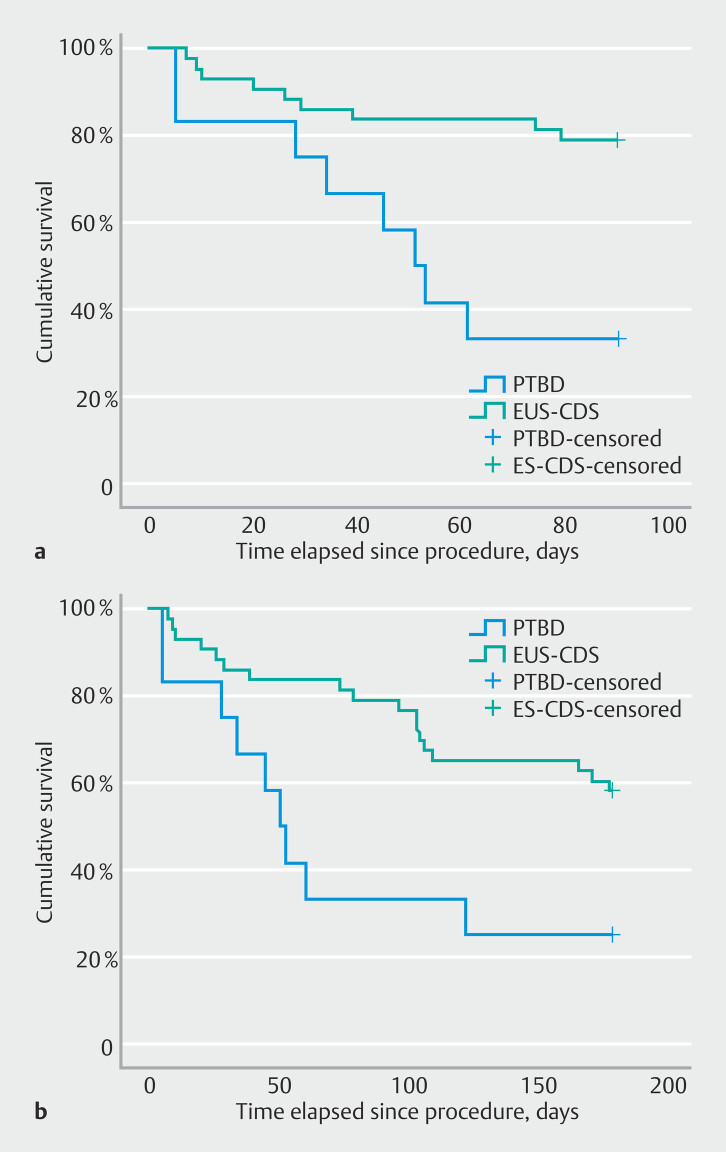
Kaplan–Meier curves of survival after percutaneous transhepatic biliary drainage (PTBD) or endoscopic ultrasound-guided choledochoduodenostomy (EUS-CDS) at:
